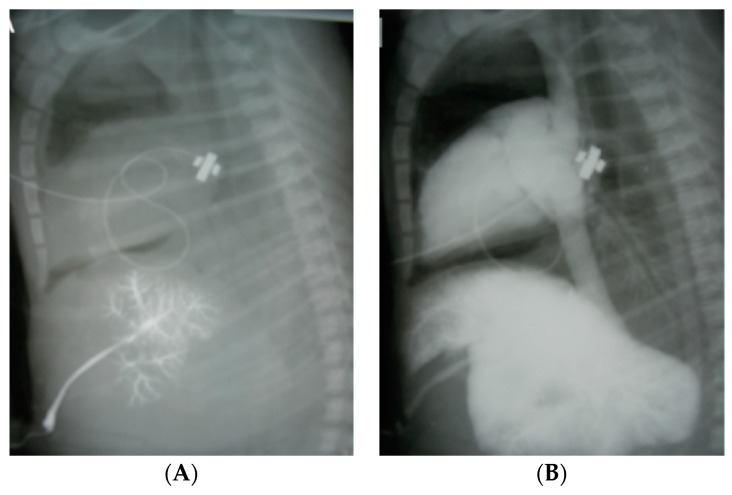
X-ray showing distribution of contrast following low- and high-volume flush through a low-lying umbilical venous catheter following 30 sec of chest compressions in term cardiac-arrested lambs. (A) Administration of 1 mL flush of Omnipaque shows the contrast remained in the portal venous system. (B) Increasing the Omipaque flush solution to 10 mL resulted in a much better distribution of contrast into the heart and great vessels. Copyright Satyan Lakshminrusimha.
